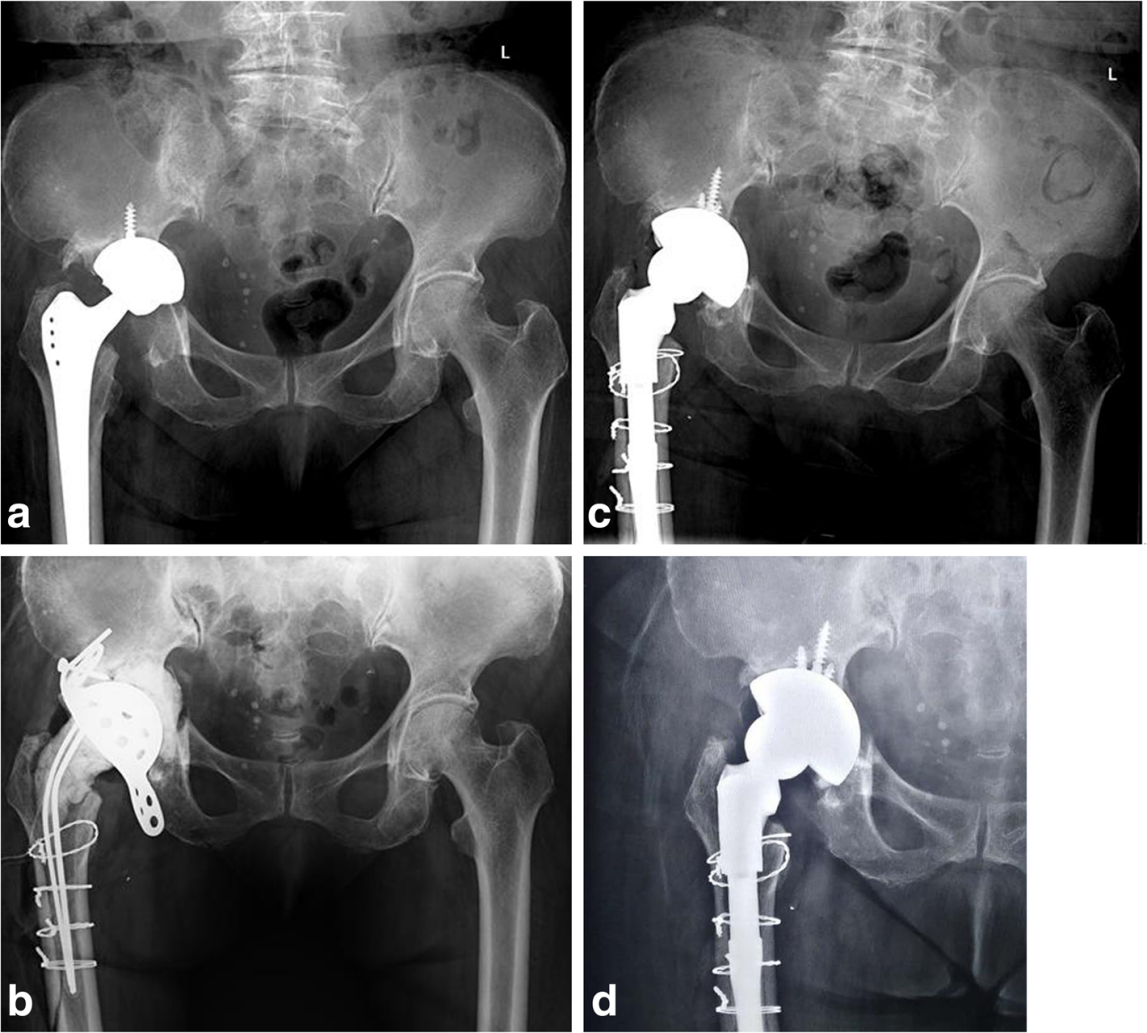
Serial radiographs of a patient of chronic pelvic discontinuity after periprosthetic joint infection. a Preoperative X-ray. b Implantation of a temporary spacer and anti-protrusion ring. c Immediate X-ray after second-stage reimplantation. d X-ray at 1 year after surgery
